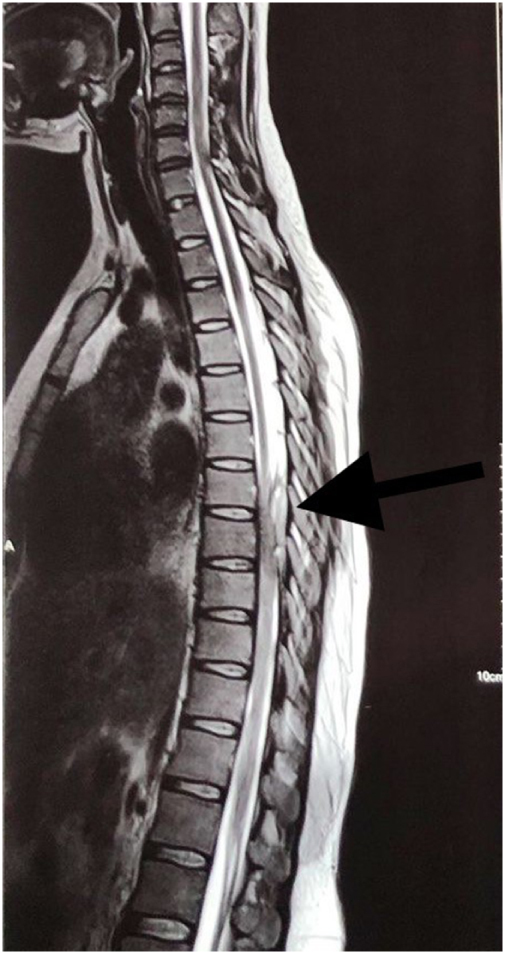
Sagittal T2-weighted MRI of the spine shows a posterior extradural lesion at the T7–T9 level, producing severe spinal canal stenosis with anterior displacement of the spinal cord. Source: Author’s own image. Published with written informed consent from the patient.
